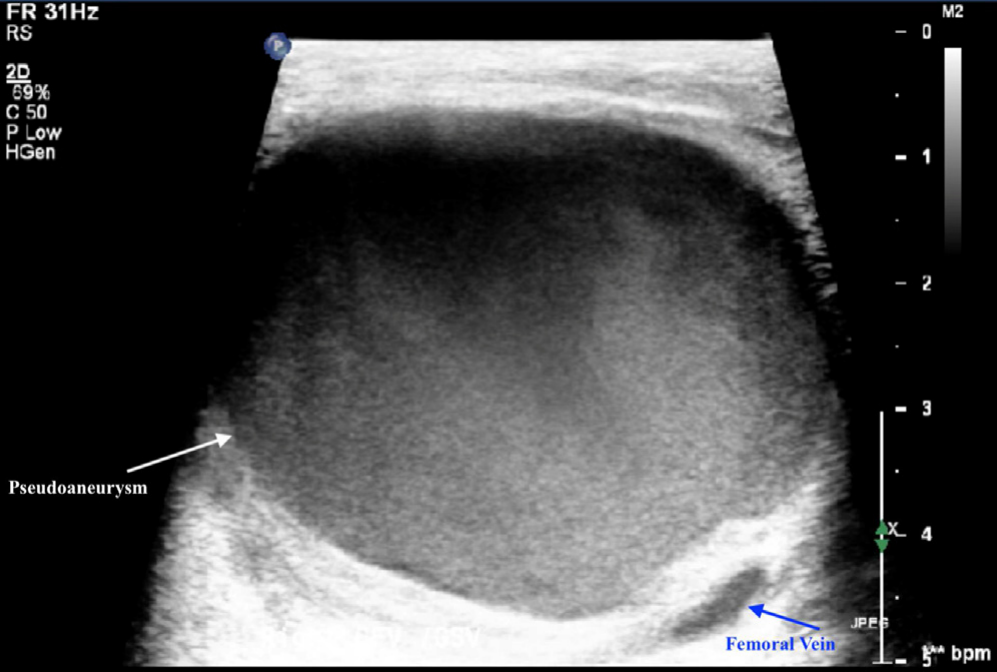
Venous duplex ultrasound showing pseudoaneurysm and femoral vein.
